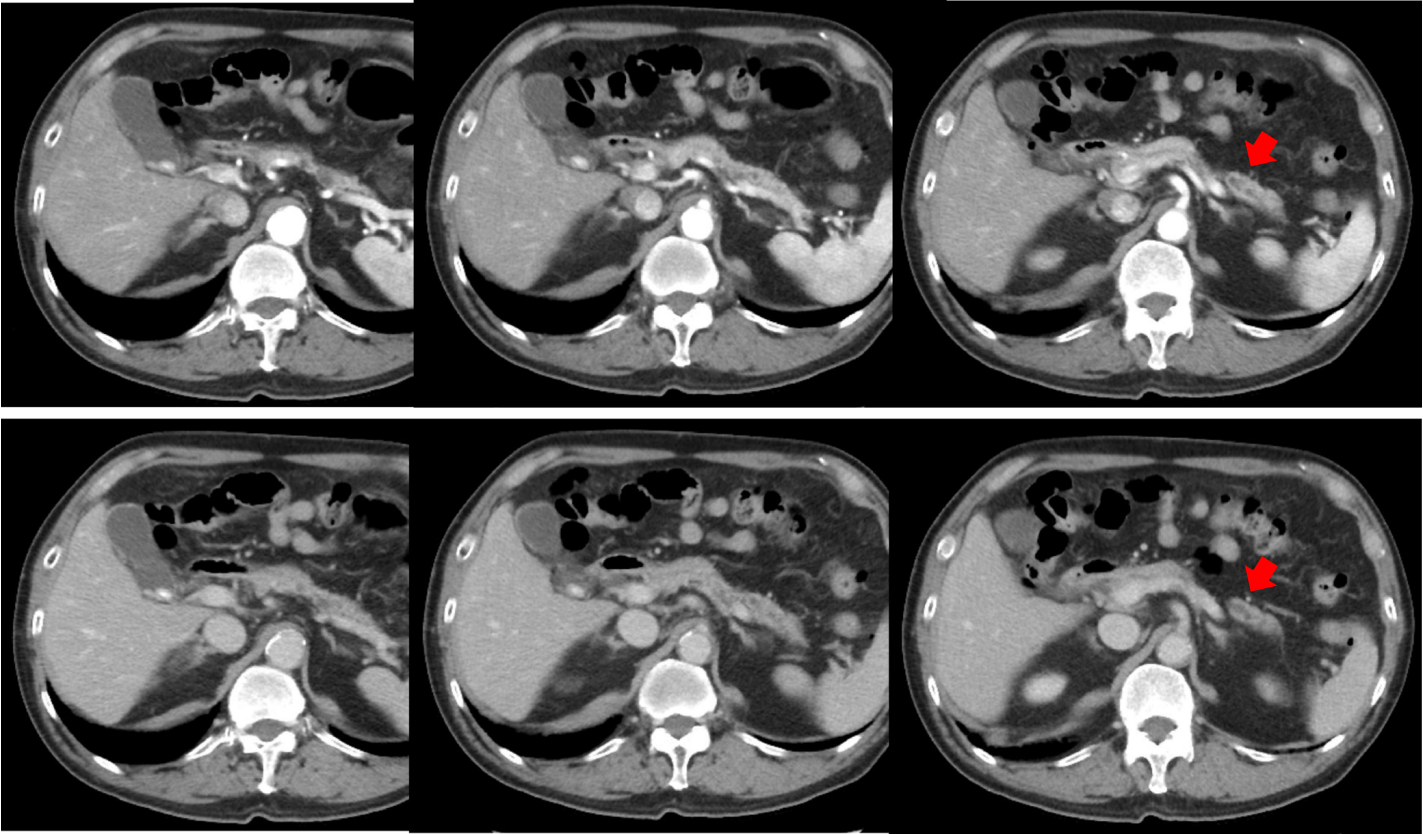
Computed tomography. The main pancreatic duct in the body and tail of the pancreas is dilated, but there is no visible tumor
